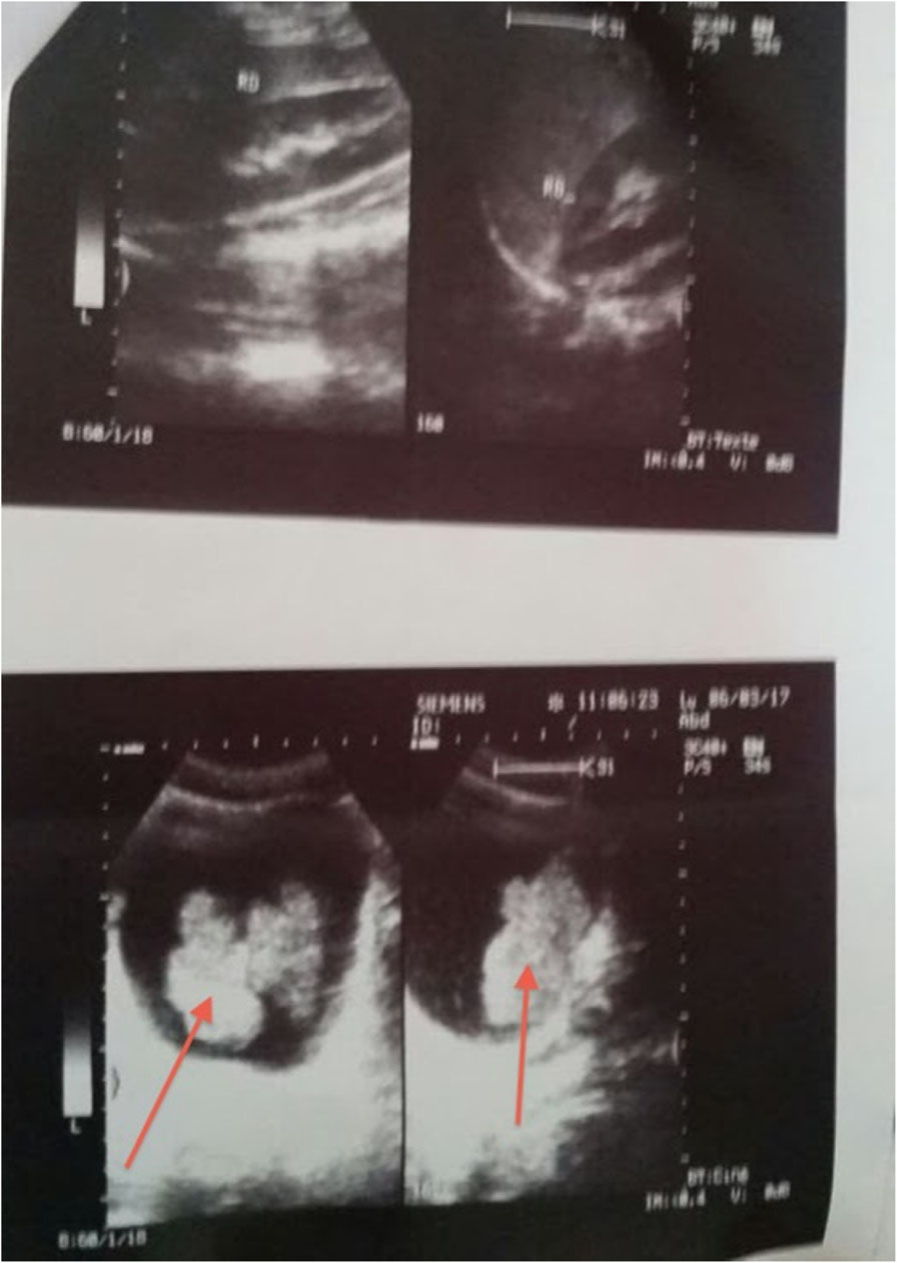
Abdomen sonography revealed a huge bladder tumor mass (70 × 60 mm, *red arrow*) at posterior wall without hydronephrosis
